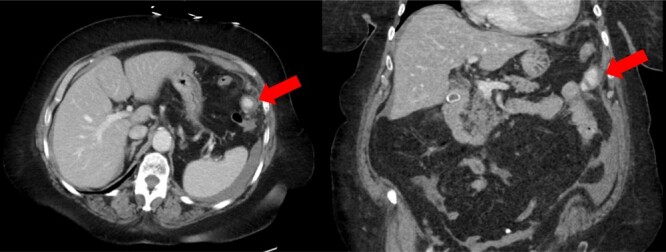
CT scan showing enhancing pseudoaneurysm (red arrow) with associated hemoperitoneum around the spleen, liver, and in left the paracolic gutter.
